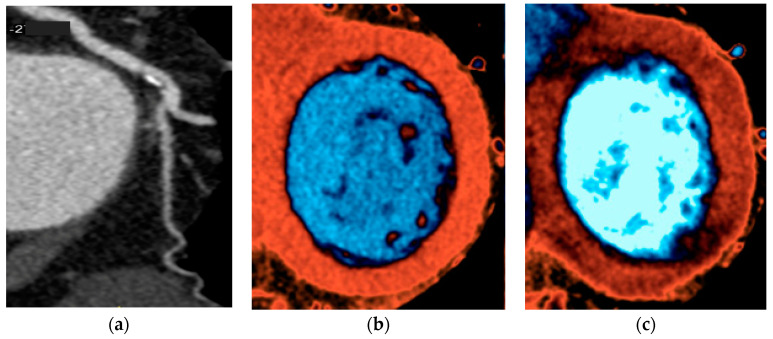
D.R. 45 years-old, female; hypertension; atypical chest pain; CCTA (a): fibrocalcific plaque in left circumflex artery with moderate stenosis; stress-CTP perfusion sequences (c) matched with rest perfusion sequences (b): reversible perfusion defect in basal inferolateral wall. CCTA: coronary computed tomography angiography; CTP: computed tomography perfusion.
